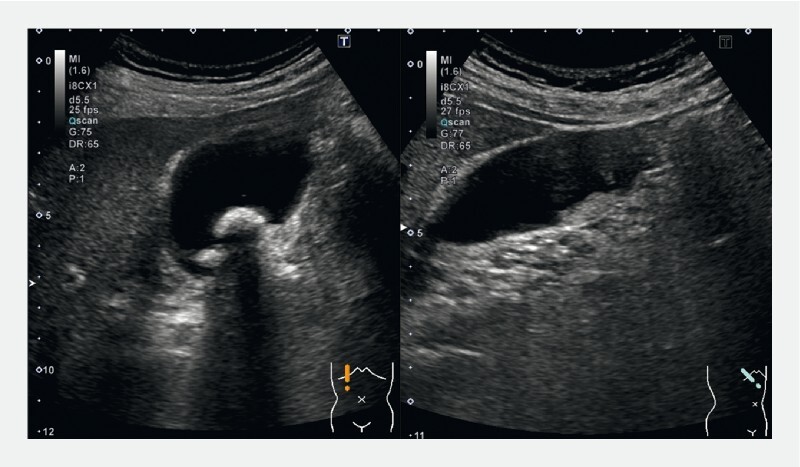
Abdominal ultrasonography revealed gallbladder stones and localized wall thickening of the gallbladder fundus.
